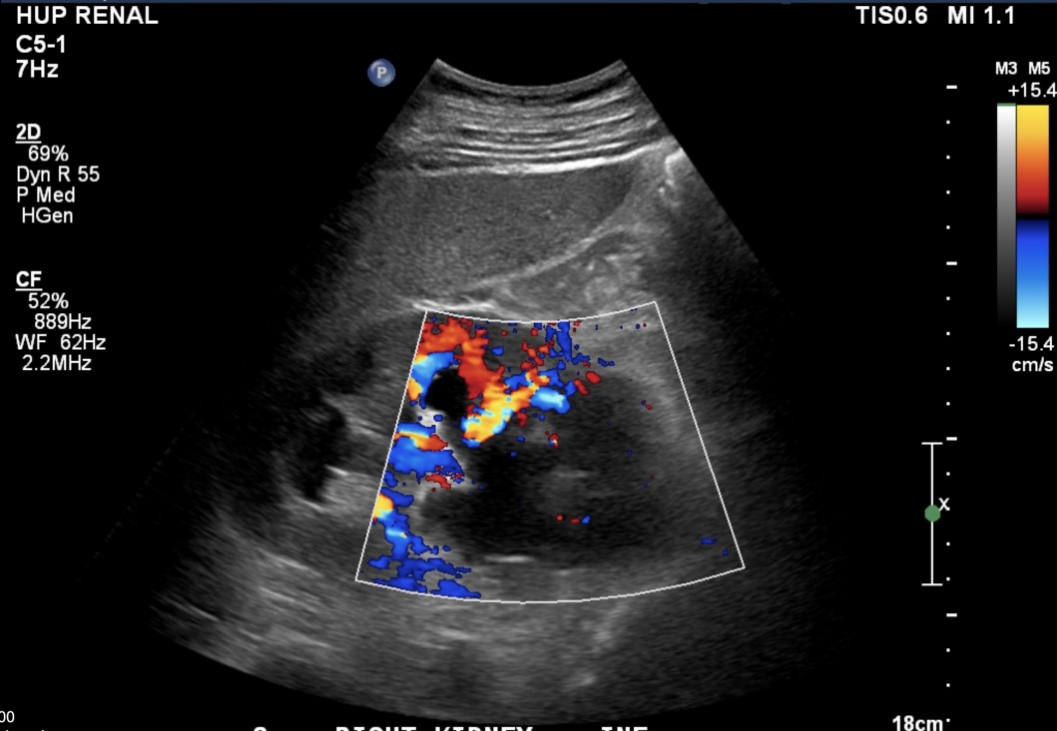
Doppler imaging of right kidney, long axis demonstrating absence of flow in the dilated renal pelvis, proximal ureter, and masses in the retroperitoneum at the inferior pole.
